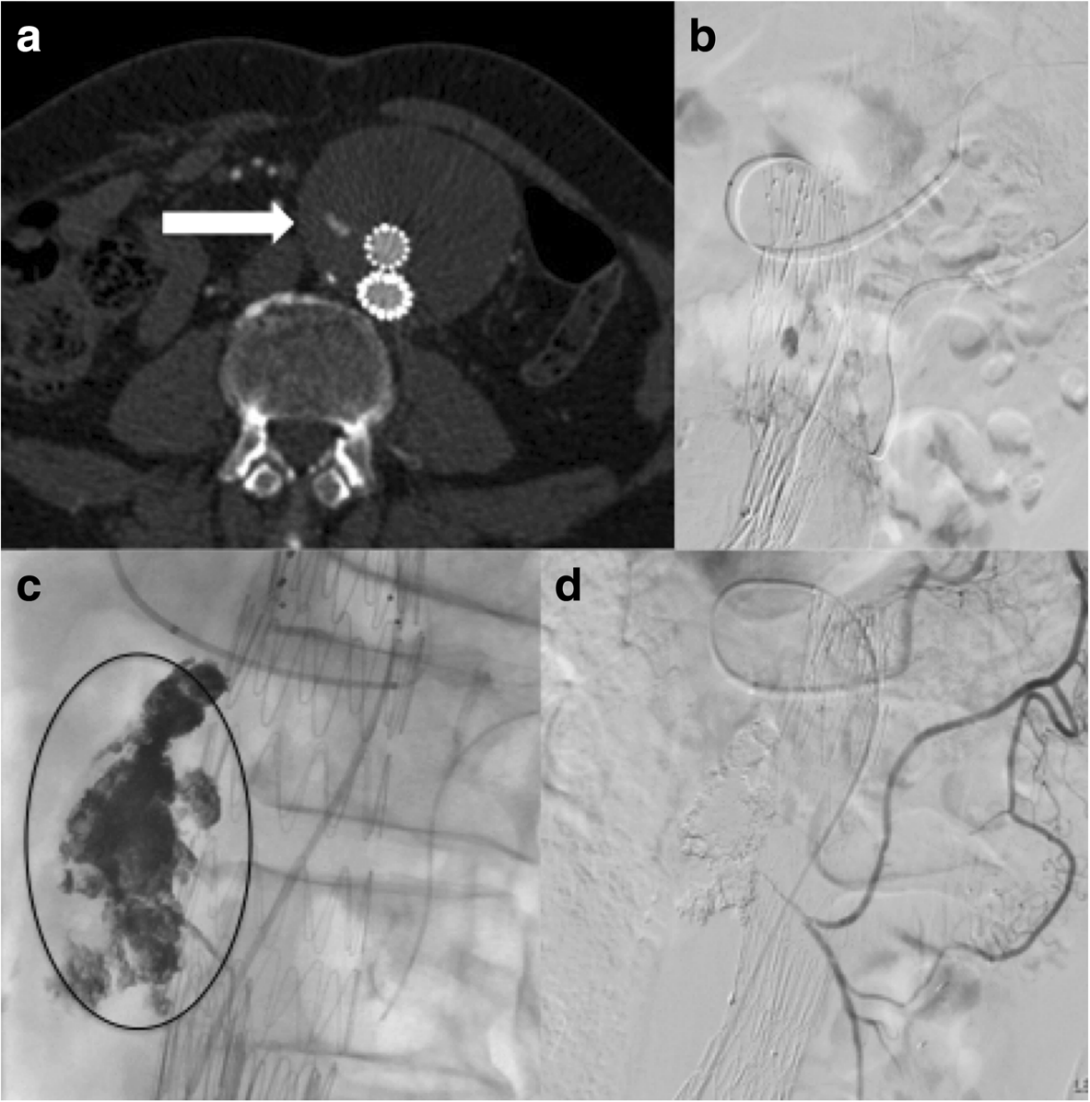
Percutaneous embolisation for type II endoleak. a Delayed CTA reveals the presence a type II endoleak (arrow). b .Angiogram confirming the access via the Riolan arcade to the IMA. c Embolisation with Onyx and (d) satisfactory angiographic result
